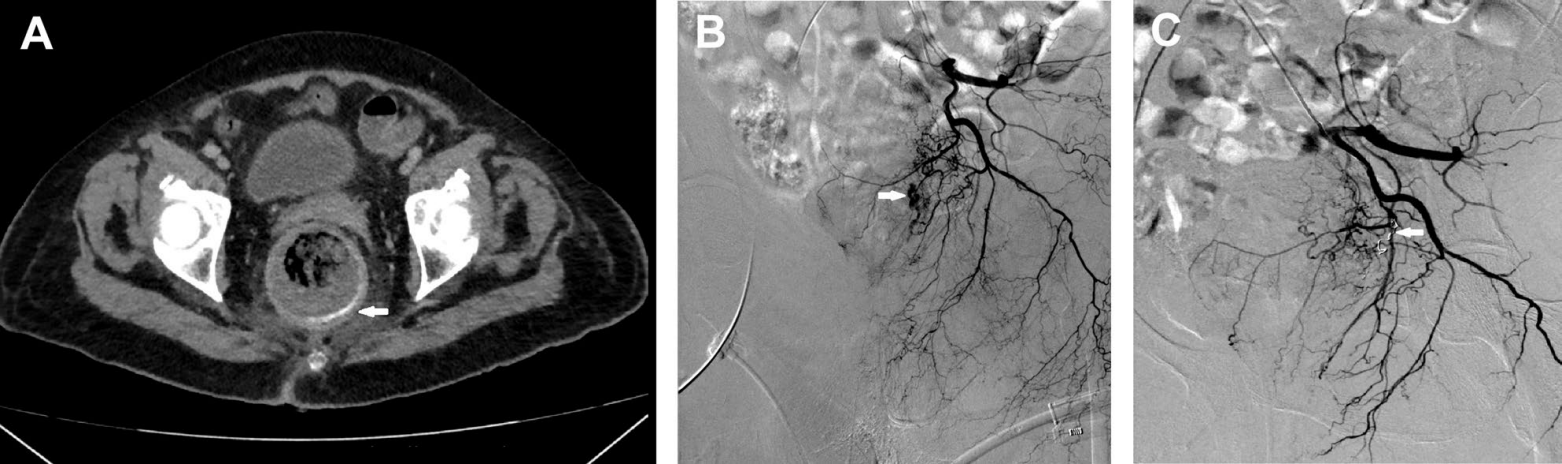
Superselective coil embolization of a middle rectal artery branch in a patient with LGIB. A Late-phase CTA image showing contrast pooling (white arrow) in the rectal lumen. B Left internal iliac artery angiogram demonstrating contrast extravasation (white arrow) from a middle rectal artery branch. C Control angiogram after superselective catheterization and coil embolization (white arrow) of the affected branch, showing no signs of bleeding
